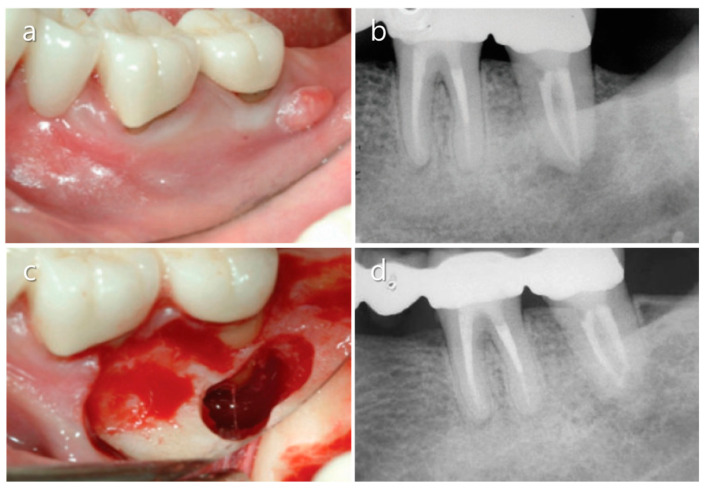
Endodontic surgery of a mandibular second molar. (a) Sinus tract formed on the buccal aspect of a left mandibular second molar. (b) Diagnostic periapical X-ray image showing that the tooth was an abutment for a fixed prosthodontic restoration. (c) The root apex was exposed after osteotomy. (d) A five-year follow-up radiograph shows complete bony healing. Images were modified from Song [31] after receiving permission from the journal.
